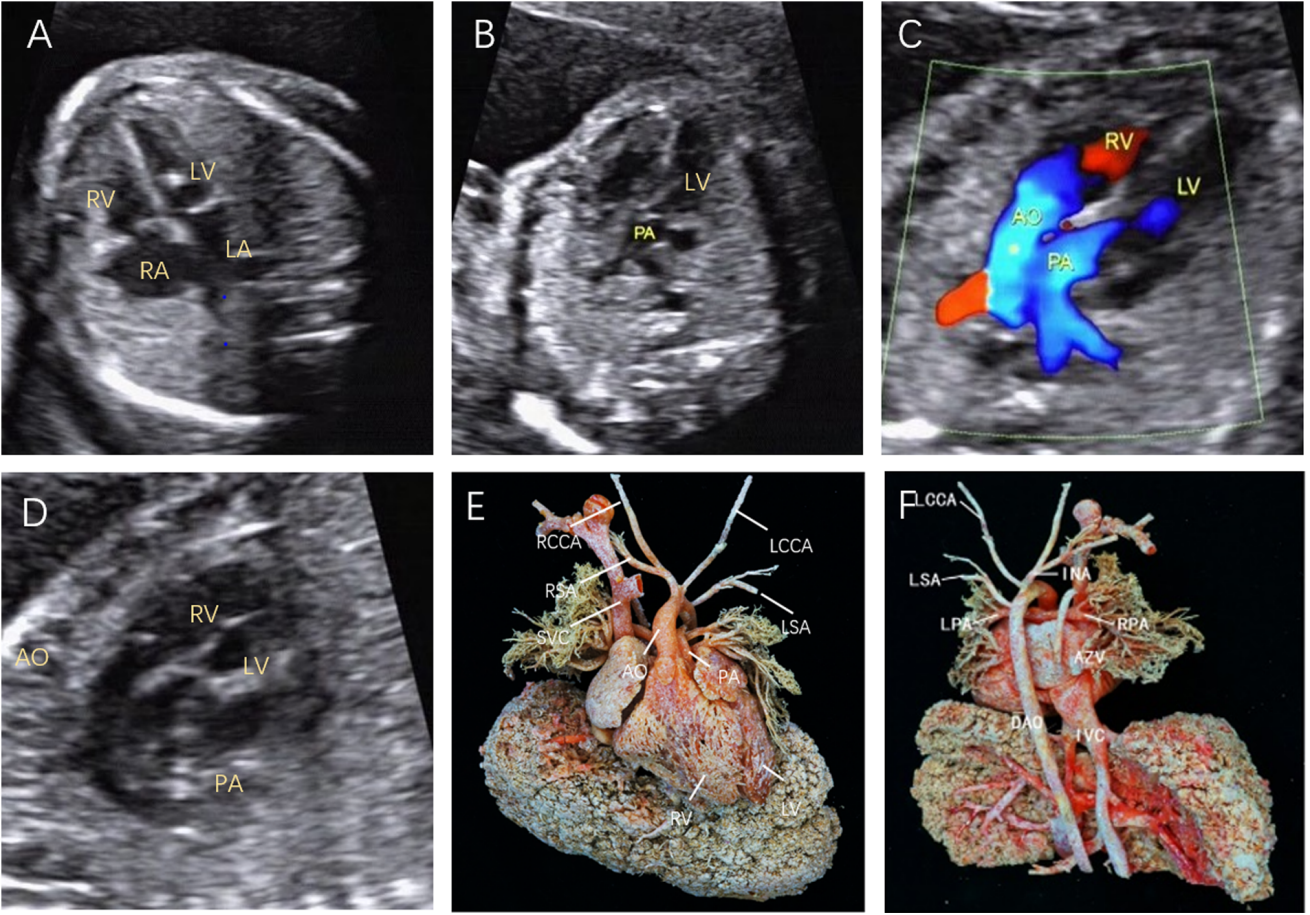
Fetal D-TGA was assessed via echocardiography at 23 weeks and 1 day. (A) Four-chamber view with consistent atrioventricular connections and strong dotted echogenicity of the left ventricle. (B) Five-chamber view showing the pulmonary artery emanating from the left ventricle and bifurcating into the left and right pulmonary arteries a short distance from the beginning of the artery. (C) Two large arteries aligned in parallel, with the aorta emanating from the right ventricle and located anteriorly to the pulmonary artery. (D) Long-axis view of the great vessels: the aortic arch emanates from the anterior right ventricle, followed by the head and neck vessels, which curl backwards in a ‘hockey puck’ shape, and the pulmonary artery follows a ‘crutch’ shape in the long-axis view. (E) Anatomical cast: frontal view. F: anatomical cast: dorsal view.
